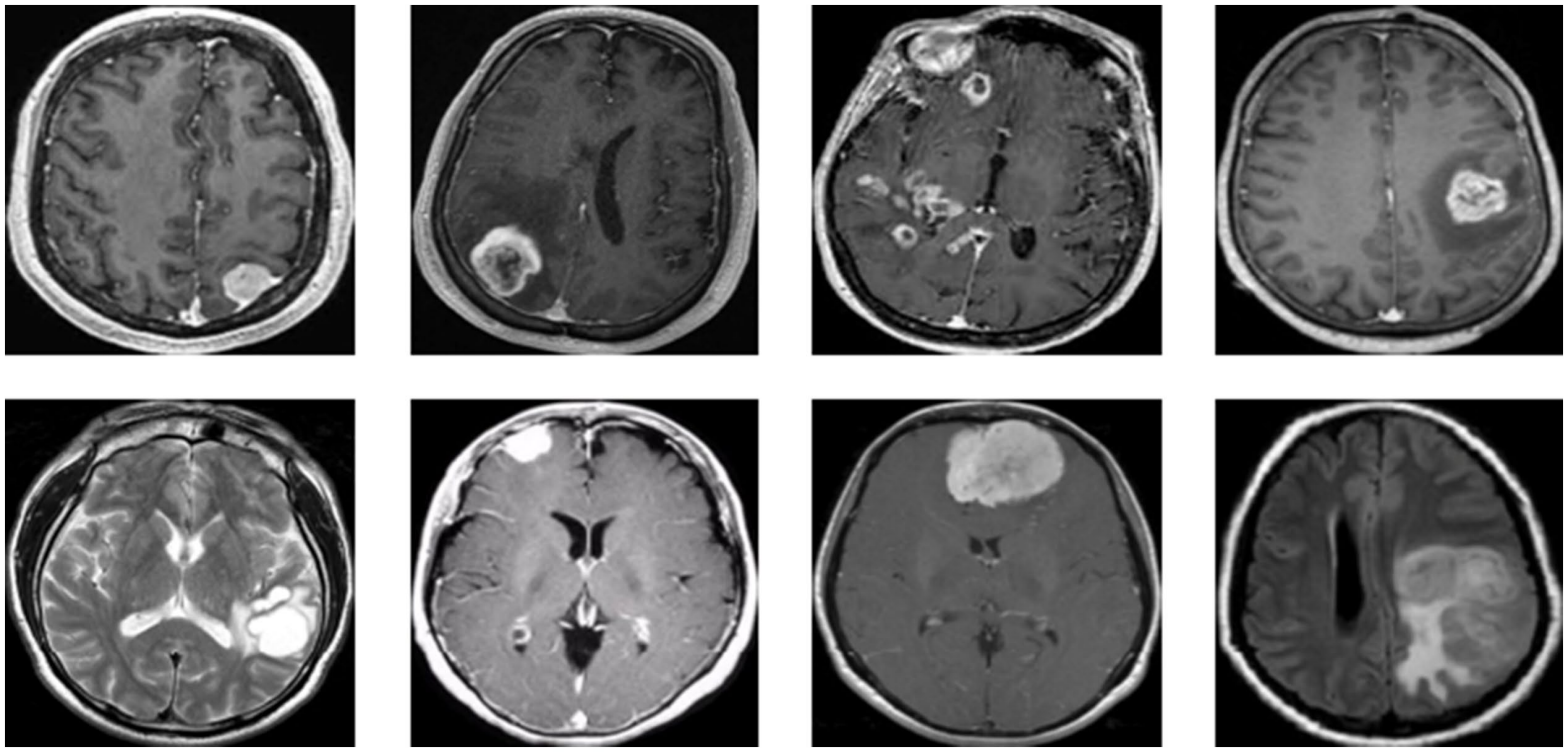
Visualization for tumor MRI scan.
